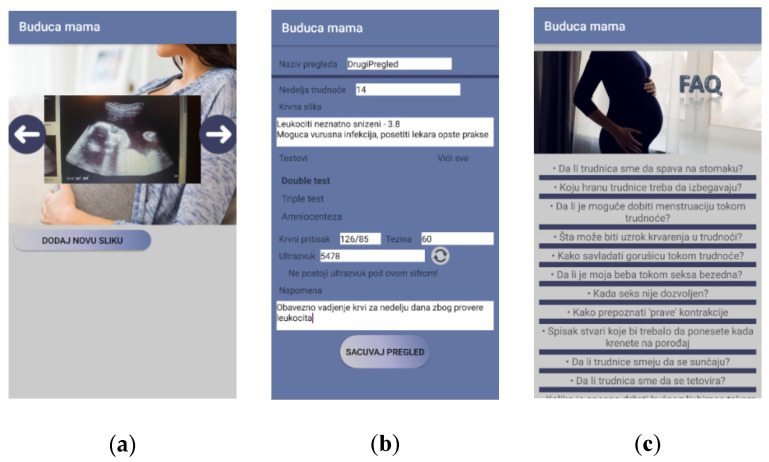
(a) Image from ultrasound device; (b) Data from ultrasound device; (c) FAQ.
